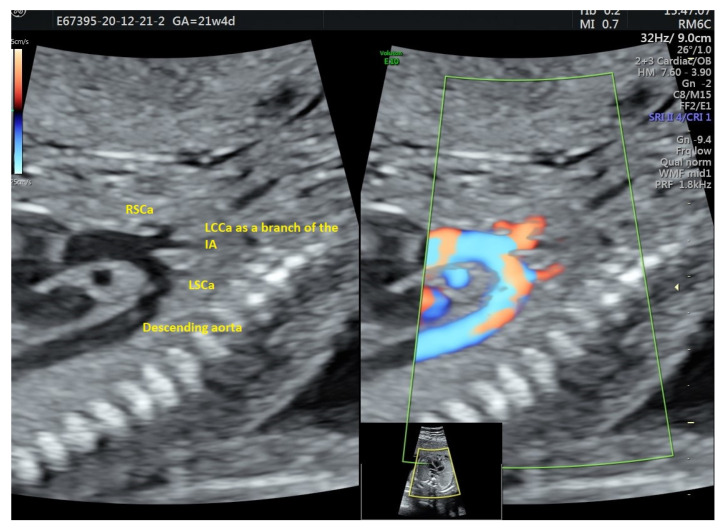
AAAV type A at 21 + 4 weeks, b mode and Doppler color, the common origin of IA and LCCa is more obvious on Doppler mode.
